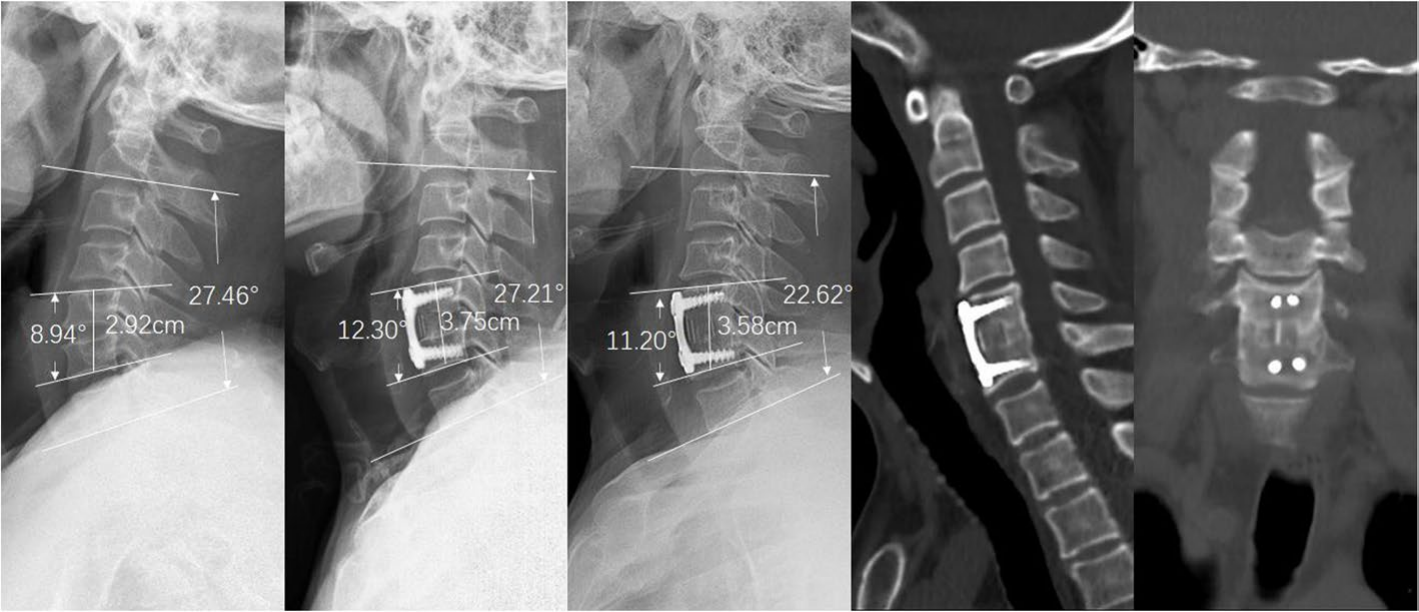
A case of a 50-year-old female patient with cervical myelopathy due to C5/6 disc herniation. a Preoperative lateral radiograph of the cervical spine showing narrowing of the disc space between C5/6. b Postoperative lateral radiograph shows that the improved n-HA/PA66 cage increased the SA and IH. c The 6-year follow-up radiograph showed no subsidence, and the SA remained stable. d, e Three-dimensional CT at the 6-year follow-up time shows grade 5 fusion. CT, computed tomography; IH, interbody height; n-HA/PA66, nanohydroxyapatite/polyamide66; SA, segmental lordosis
